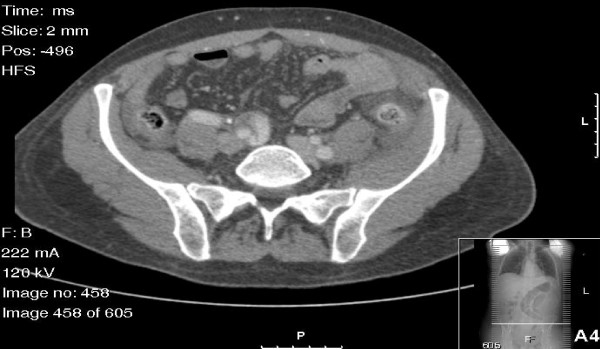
Computed tomographic scan demonstrating the right common iliac artery aneurysm (2.7 cm in diameter) with dissection. The left common iliac artery measures 1.4 cm.
